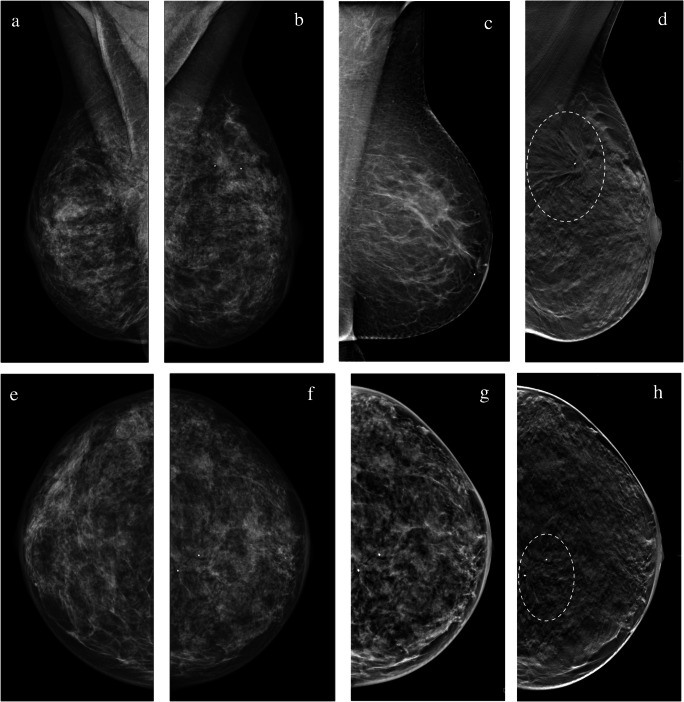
A 51-year-old woman with extremely dense breasts. An architectural distortion was visible in the upper quadrant of the left breast (circle). The lesion was detected and classified as probably benign on digital mammography (DM) by one in four readers and suspicious by the other three readers. All four readers agreed in identifying the lesion as suspicious when evaluating one-view wide-angle digital breast tomosynthesis with synthetic mammography (WA-DBT with SM) and two-view WA-DBT with SM. The lesion was biopsied under ultrasound guidance and the diagnosis was fibrotic breast tissue with multiple papillomas. The absence of malignancy was confirmed at surgery. (a, b DM bilateral mediolateral oblique view (MLO); c SM MLO; d DBT MLO; e, f DM bilateral cranio-caudal view (CC); g SM CC; h DBT CC)
